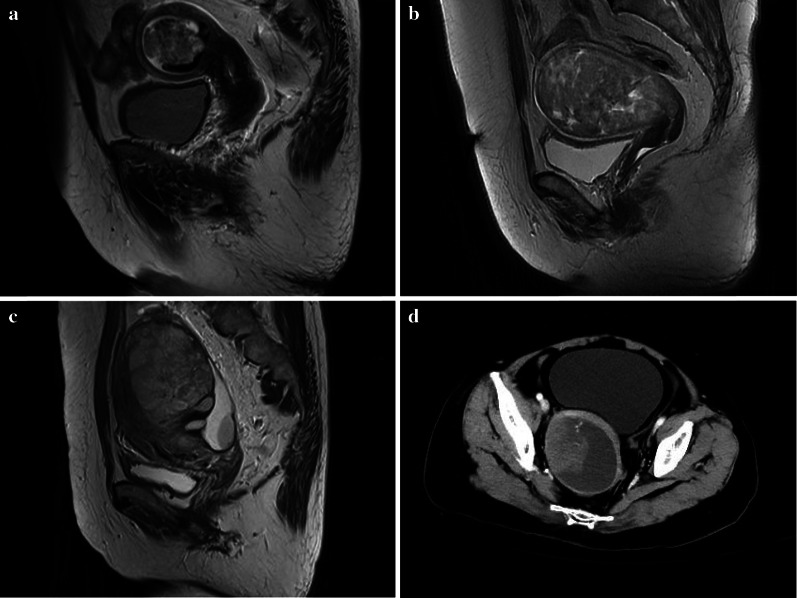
Images of uterine carcinosarcoma (UCS). Sagittal T2WI magnetic resonance imaging (MRI) of a 64-year-old woman (a) showed a mixed signal tumor in the cavity with multiple small round or irregular cystic areas in the internal tumor. Sagittal T2WI MRI of a 66-year-old woman (b) showed a mixed signal tumor in the cavity with many gap-like cystic areas. Sagittal T2WI MRI of a 49-year-old woman (c) showed a high signal tumor in the cavity with many oval cystic areas. Axial computed tomography (CT) images in the venous phase (d) of a 66-year-old woman showed a tumor mainly composed of cystic components. The tissue components showed nipple-like enhancement with a marginal cystic component
